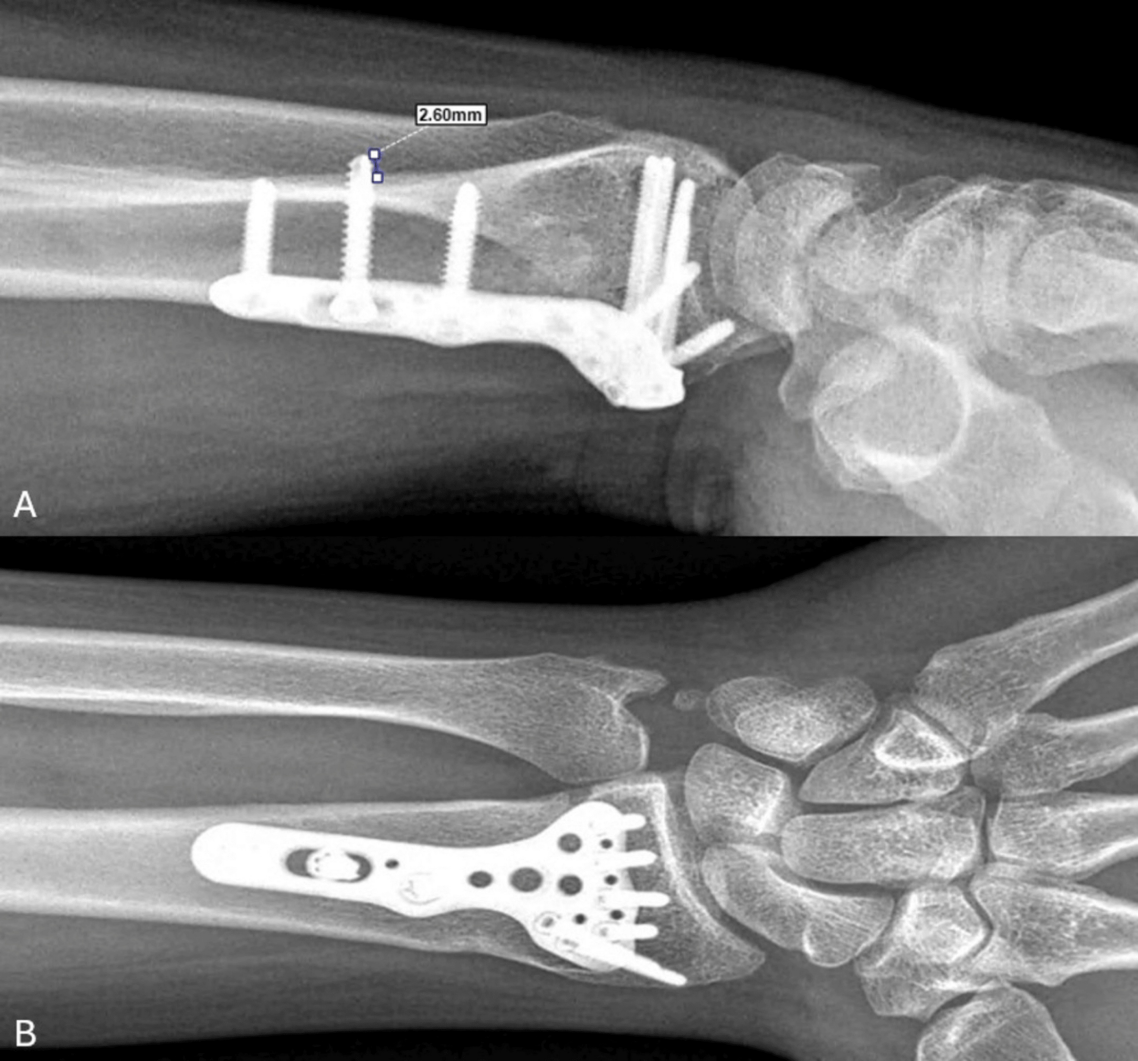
Postoperative 15-month wrist X-ray of a 41-year-old male patient. (A) Measurement of the dorsal radius cortex with the most prominent screw on a lateral direct radiograph of a healed distal radius fracture treated with a volar plate. (B) Posteroanterior wrist X-ray of the same patient.
